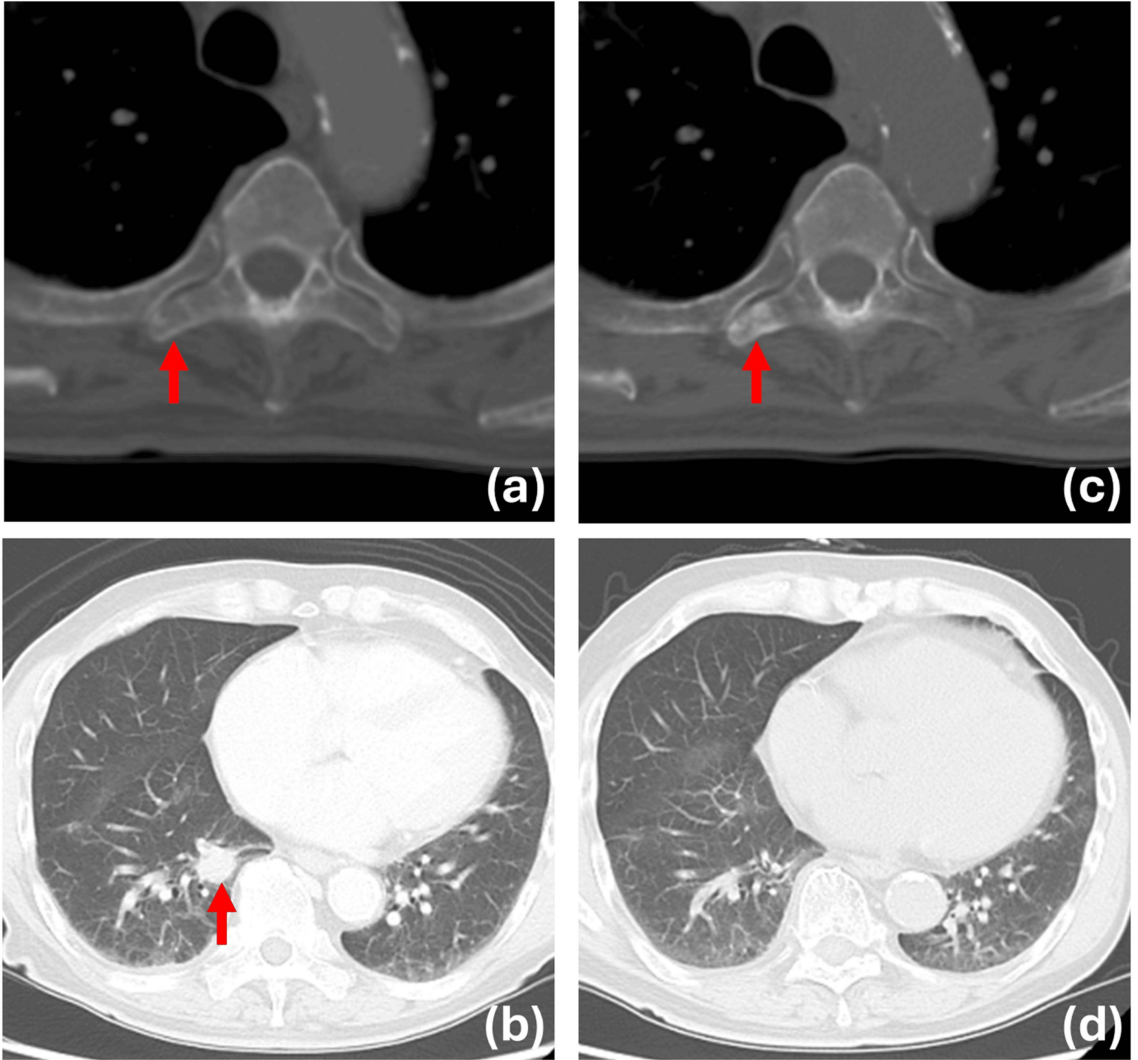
CT findings of the present patient. (a) CT of the thoracic vertebrae before pembrolizumab administration. Osteolytic changes were observed in the right transverse processes of the fifth thoracic vertebra (red arrow), where positron emission tomography-computed tomography (PET-CT) demonstrated accumulation. (b) CT of the lungs before pembrolizumab administration. An 18-mm nodule was found in the right middle lobe (red arrow). (c) CT at month five after the first pembrolizumab administration. The osteolytic changes in the right transverse processes of the fifth thoracic vertebra demonstrated signs of osteosclerosis (red arrow). (d) CT of the lungs at month seven after pembrolizumab administration demonstrated complete resolution of the lung metastases.
